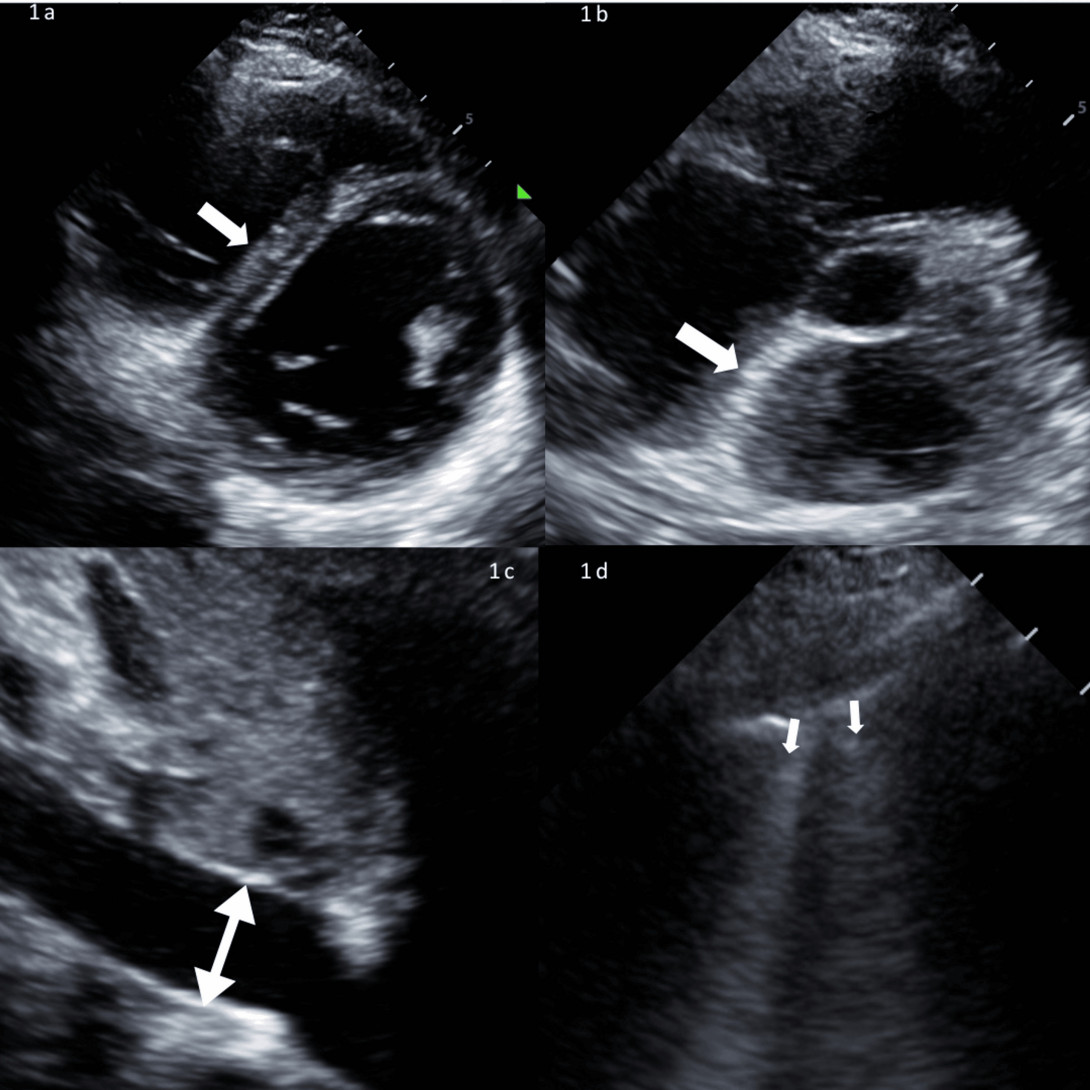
Signs of raised left atrial pressure and organ congestion 1a: Parasternal short-axis view showing flattened D-shaped interventricular septum (white arrow) with paradoxical movement in diastole. 1b: Parasternal short-axis view showing deviated interatrial septum to the right (white arrow). 1c: Inferior vena cava, subcostal view showing a dilated inferior vena cava (2.4 cm) with minimal respiratory variation (white arrow). 1d: Lung ultrasound, right upper quadrant showing B lines (white arrow).
